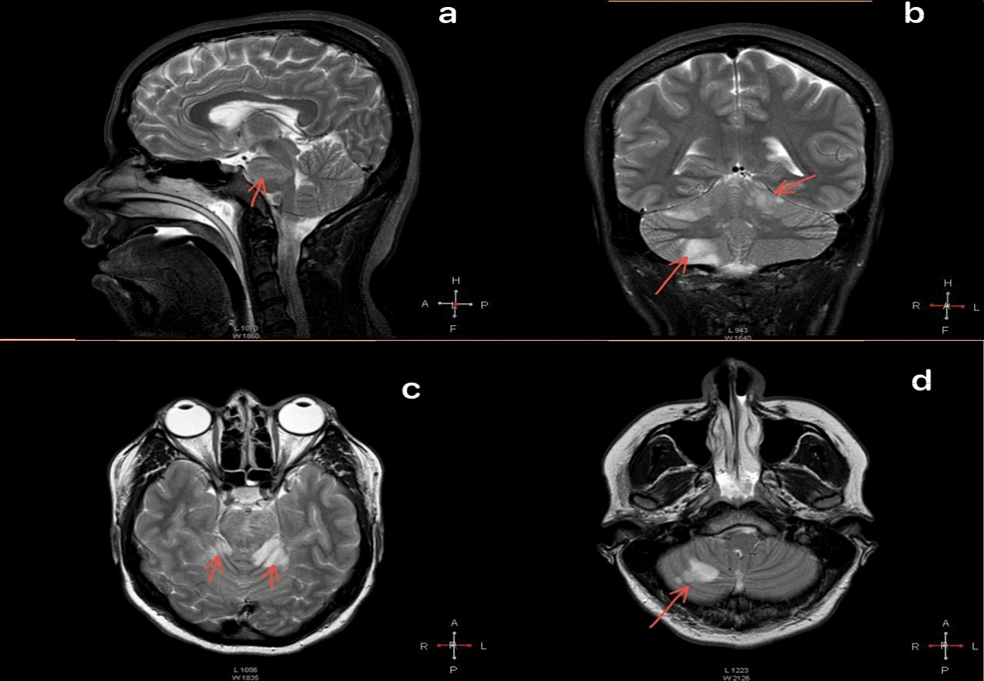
Sagittal, coronal, and axial magnetic resonance image a: Sagittal T2 magnetic resonance (MR) showing pons hyperintensity. b: Coronal T2 MR with diffuse hyperintensities in cerebellar hemispheres with more significant right extension. c: Axial T2 MR, diffuse hyperintensities in both cerebellar hemispheres and diffusely in the pons. d: Axial T2 MR showing diffuse hyperintensity in the right cerebellar hemisphere, compatible with an ischemic event of the posterior circulation.
